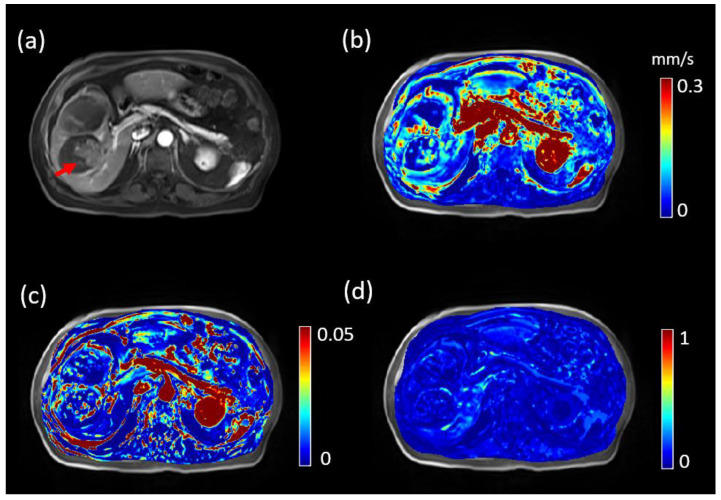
DCE MRI, QTM velocity, $K^{trans}$ and $V_{e}$ maps of HCC of a 78-year-old patient with lung shunting fraction 15.3%. (a) post-Gd T1 weighted image showing the tumor (red arrow). (b) QTM |*u*| and $V_{e}$ map, (c) $K^{trans}$ map, and (d) $V_{e}$ map.
